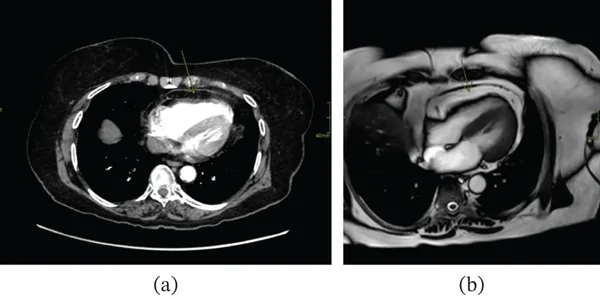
(a) Computed tomography (CT) of the chest showing findings suggestive of epicardial and mediastinal lipomatosis. (b) Cardiac MRI showing diffuse epicardial lipomatosis.
